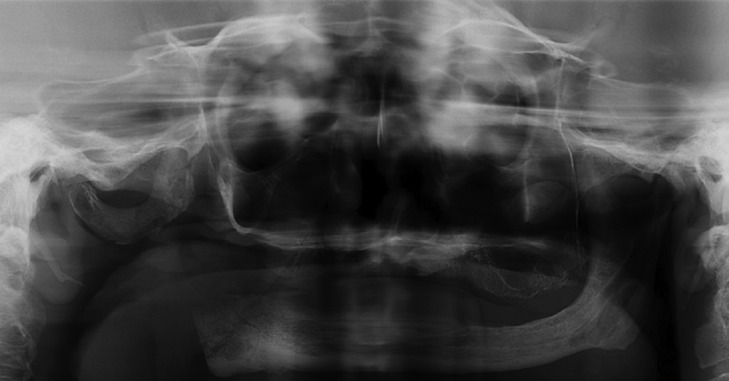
Post-operative panoramic radiography, with three-years of follow-up and no signs of tumor recurrence.
